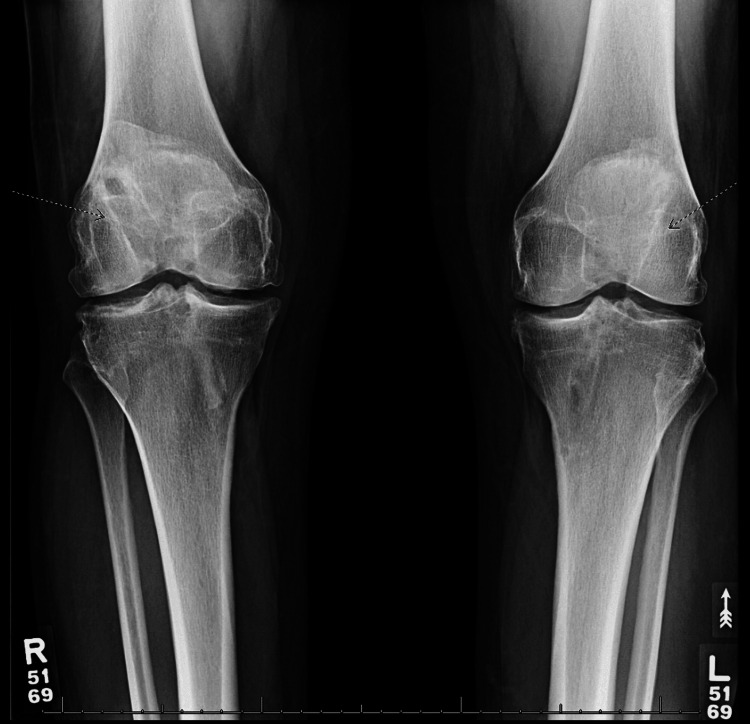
Preoperative radiographs. Bilateral anteroposterior view of the knee showing degenerative changes and vertical femoral tunnel placement (dotted arrows).
